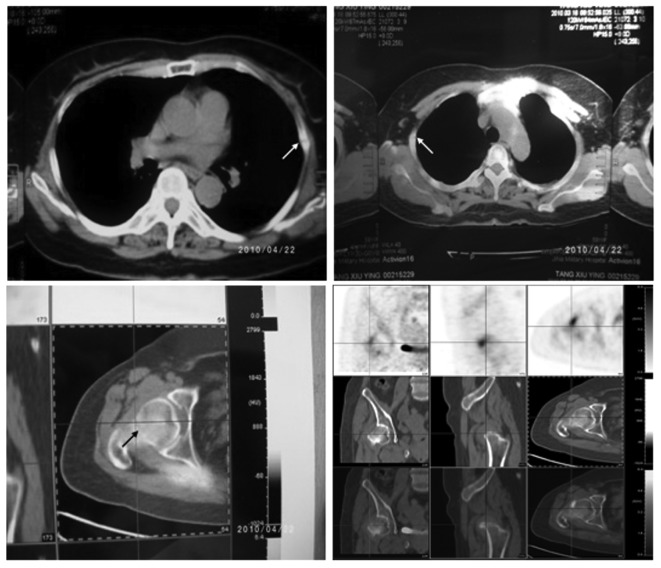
Whole body PET-CT examination. Several ribs and double femoral neck (incompletely) had continuous discontinuity and numerous old fracture lines were present (indicated by arrows indicate loose lines). PET-CT, positron-emission tomography-computed tomography.
